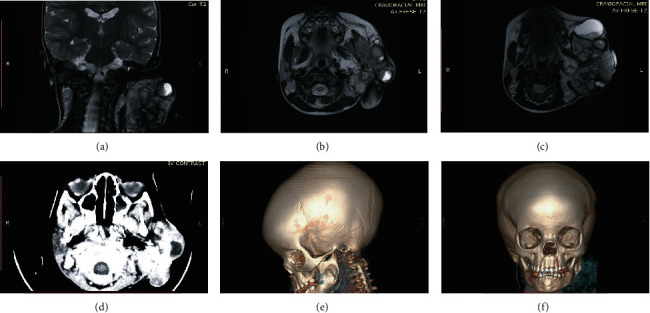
Radiologic imaging of a female child diagnosed with a *MAML2*-rearranged mucoepidermoid carcinoma of the parotid gland. MRI T2-weighted coronal and axial views (a)–(c) CT scan axial view (d), and 3D-refomatted CT scans (e, f) showing a soft tissue swelling over the left jaw region with no bony lytic or sclerotic lesion. The bony outline was preserved, with a cupping effect on the ramus of the mandible.
